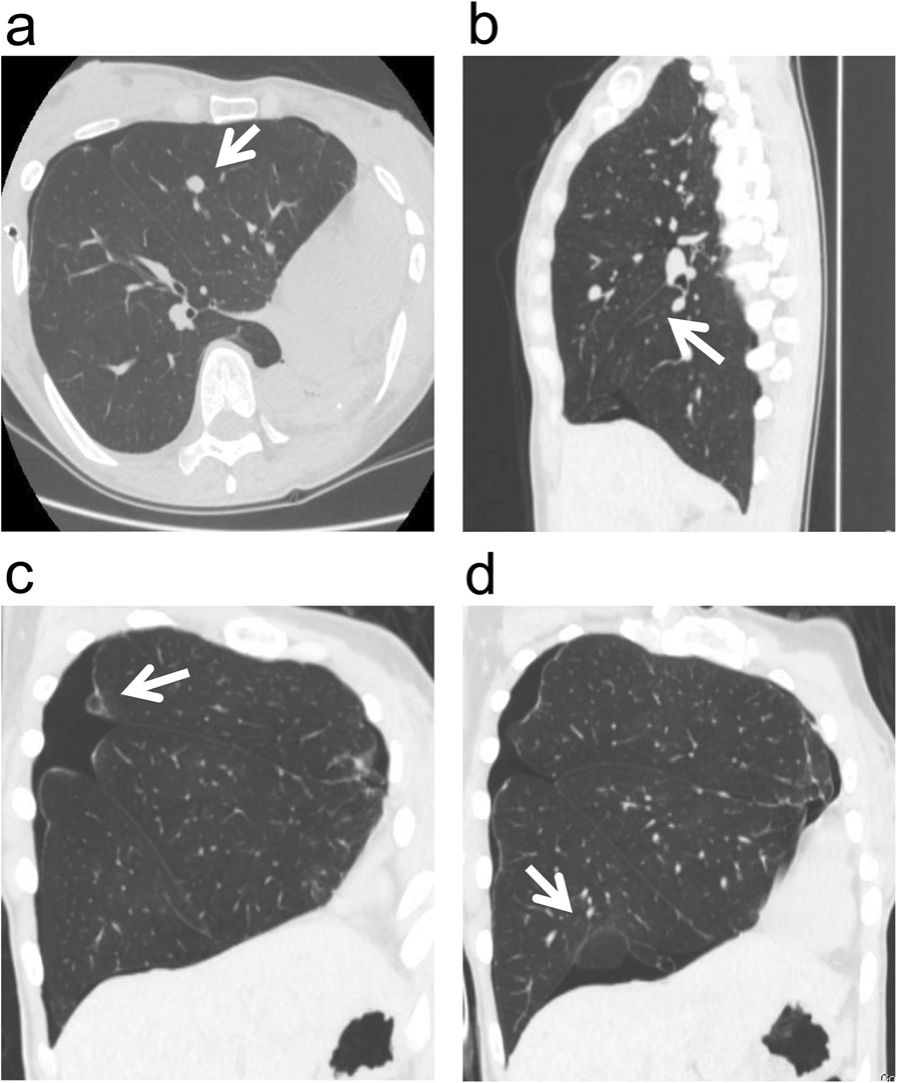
Computed tomography (CT) image of the chest. CT image (a, axial view; b, sagittal view; c and d, coronal views) reveals a tumor in the deep part of the right S4 lung segment (a and b) and bullae on the right upper and lower lobes of the lung (c and d).
